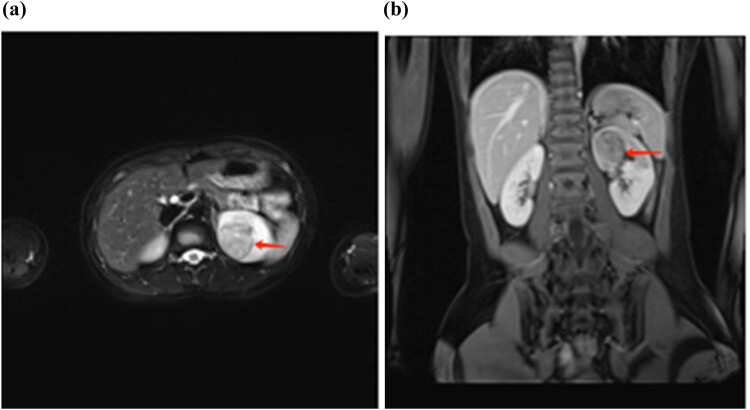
MRI image showing a solid mass in the upper pole of the kidney with long signal in T2WI: (a) transverse plane and (b) coronal plane.
